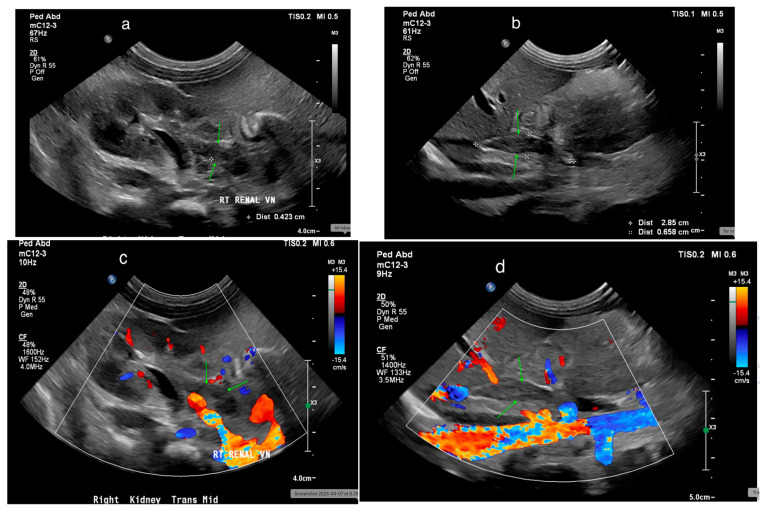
(a) Thrombosis in the renal vein (green arrows) in a 7-day-old male. (b) Thrombosis in the inferior vena cava (IVC) (green arrows) in a 7-day-old male. (c) Doppler ultrasound (US) shows minimal flow around the thrombus in the right renal vein (green arrows). (d) Doppler US shows no flow around the thrombus in the IVC (green arrows).
